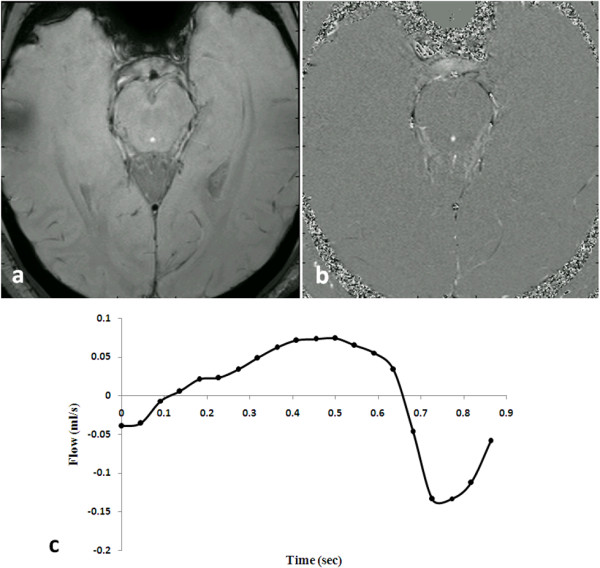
Flow in the cerebral aqueduct from cine phase contrast MRI in a healthy control. A typical MRI flow study, using the phase contrast technique, consists of the magnitude (a, anatomical) and phase (b, flow) image. In this example, cine images were taken as a function of the cardiac cycle by gating the image acquisition to a peripheral pulse signal. The image on the right depicts flow velocities during one phase of the cardiac cycle with caudal CSF flow (the bright dot in center of the image shows caudal flow in the aqueduct during this phase of the cycle). By summing over all pixels in the aqueduct, a net flow waveform is obtained (c). Stroke volume in this instance was 25.7 μl.
